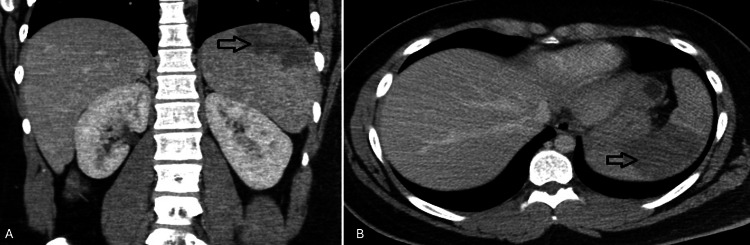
CT scan of the abdomen demonstrating splenic infarction. (A) Coronal view and (B) transverse view.
